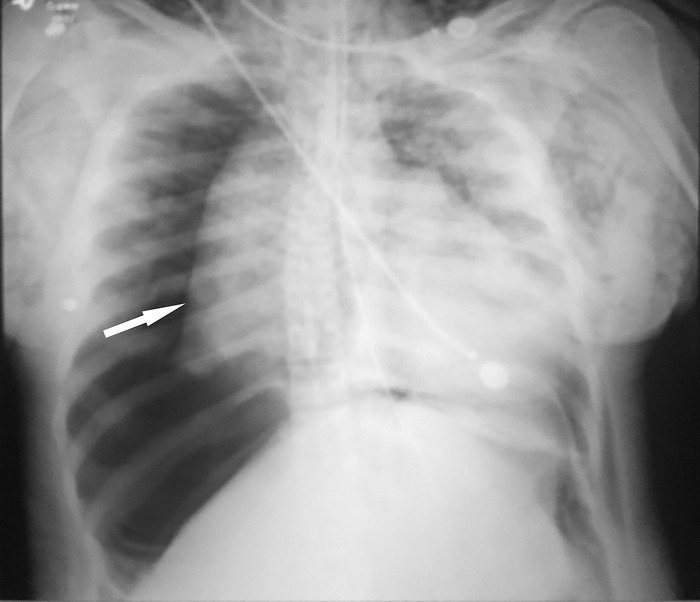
Intraoperative chest x-ray shows bilateral subcutaneous air, pneumomediastinum, and a large right-sided tension pneumothorax (white arrow).
